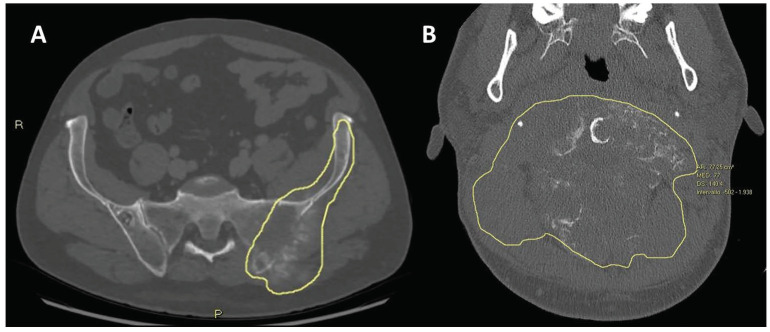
Baseline CT scans of two patients affected by osteosarcomas located in skeletal segments difficult to reach surgically, namely (A) iliac bone and (B) upper cervical spine. Tumor margins are depicted with yellow lines. Both lesions were declared inoperable by multidisciplinary tumor board from sarcoma reference center.
